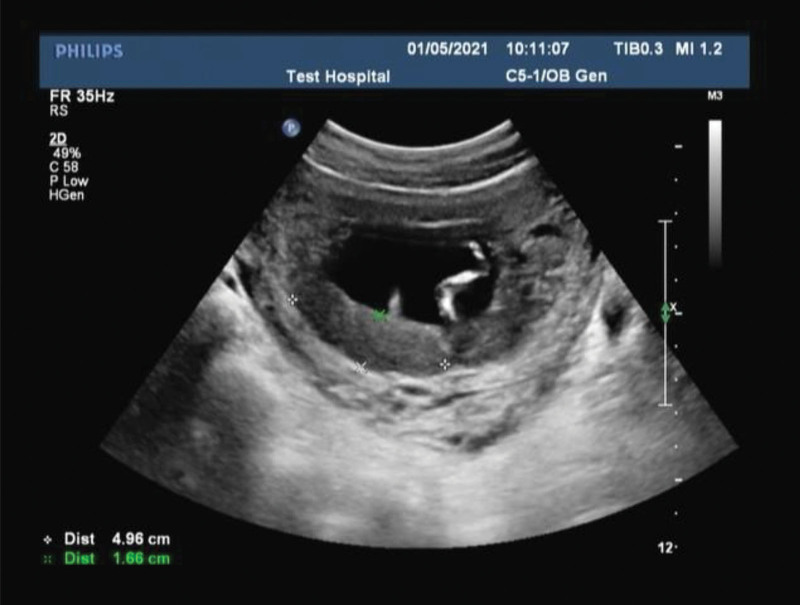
January 5, 2021: Ultrasound showed a hypoechoic region at the placenta lower edge, measuring 5.0 cm × 1.7 cm, with visible fluid movement within.
